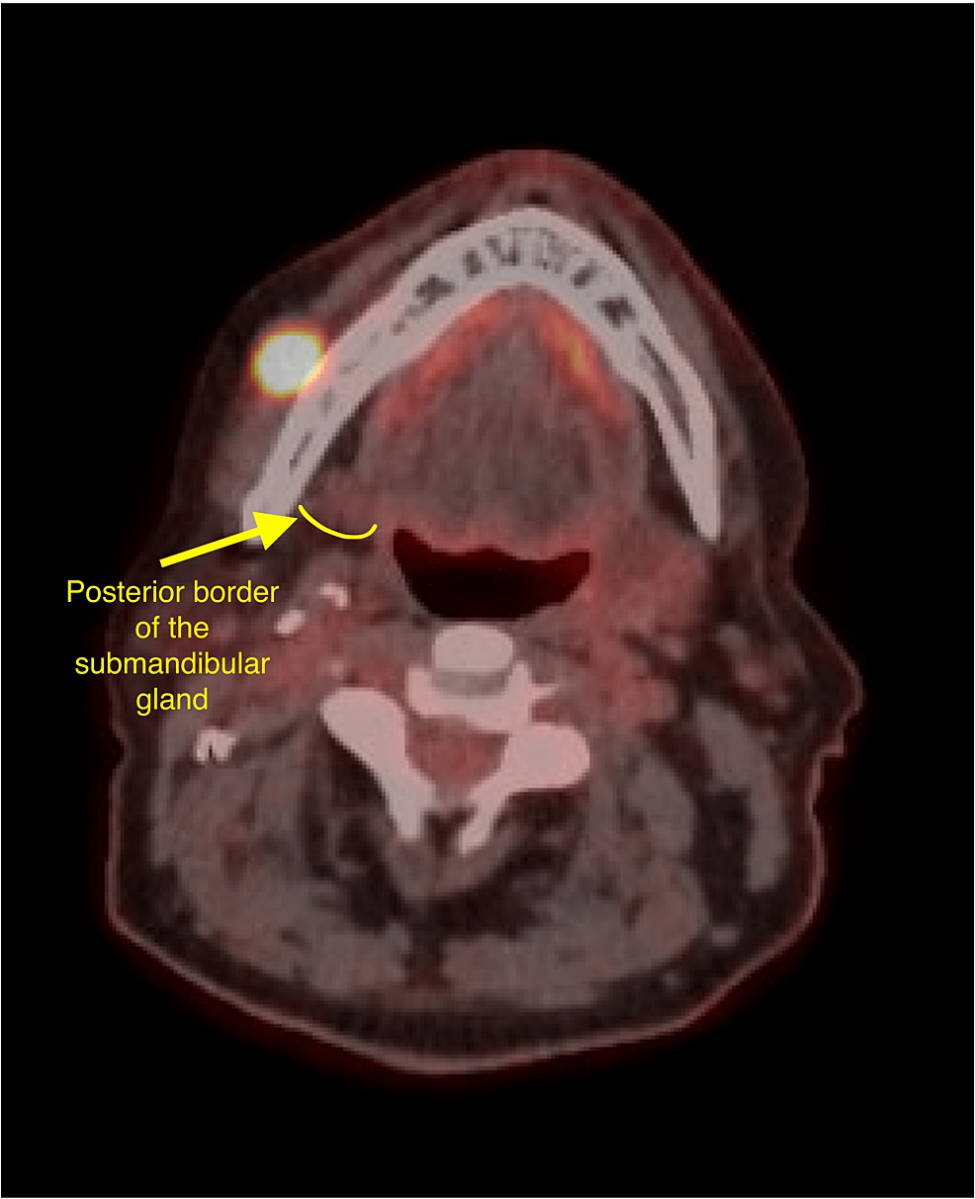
PET-CT axial view three years after initial CT showing a hypermetabolic prominent but non-enlarged level Ib lymph node (SUVmax of 14.0). PET-CT: positron emission tomography-computed tomography; CT: computed tomography; SUVmax: maximum standardized uptake value
